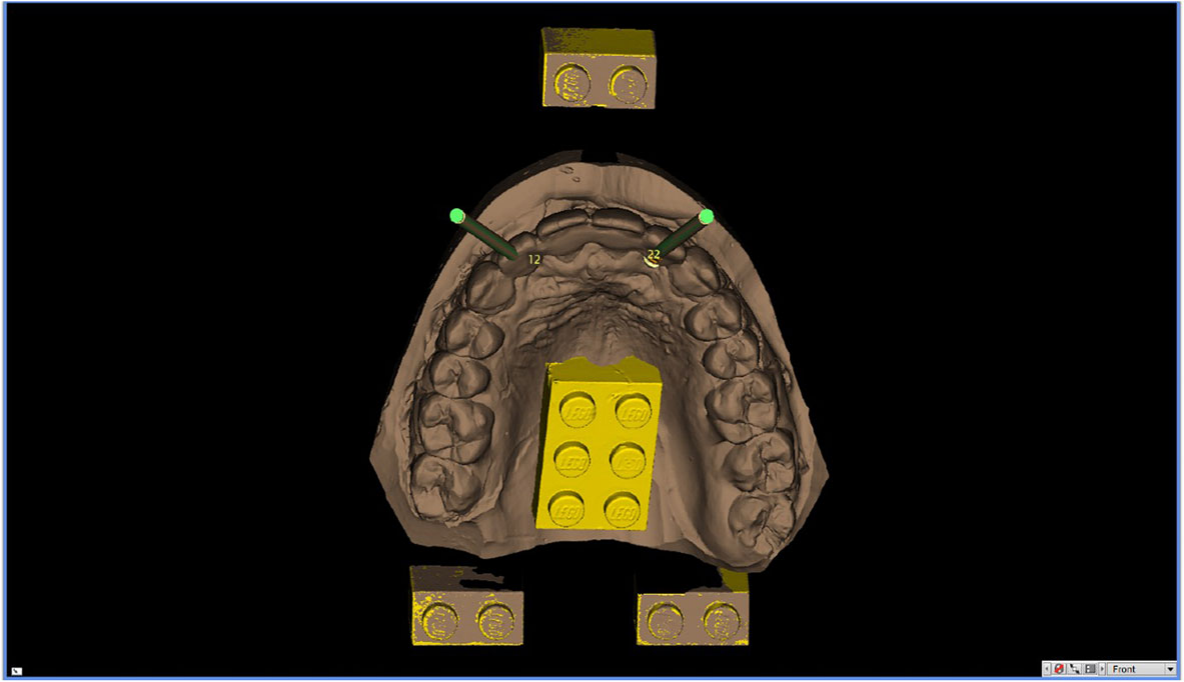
The model of the teeth is imported in the guided surgery software and superimposed on the CBCT reconstruction by means of reference points (Lego bricks). These reference points are also useful to understand the quality of the CBCT, in order to highlight any possible patient movement and possible related CBCT distorsion. The superimposition, by points and surfaces, is extremely accurate
